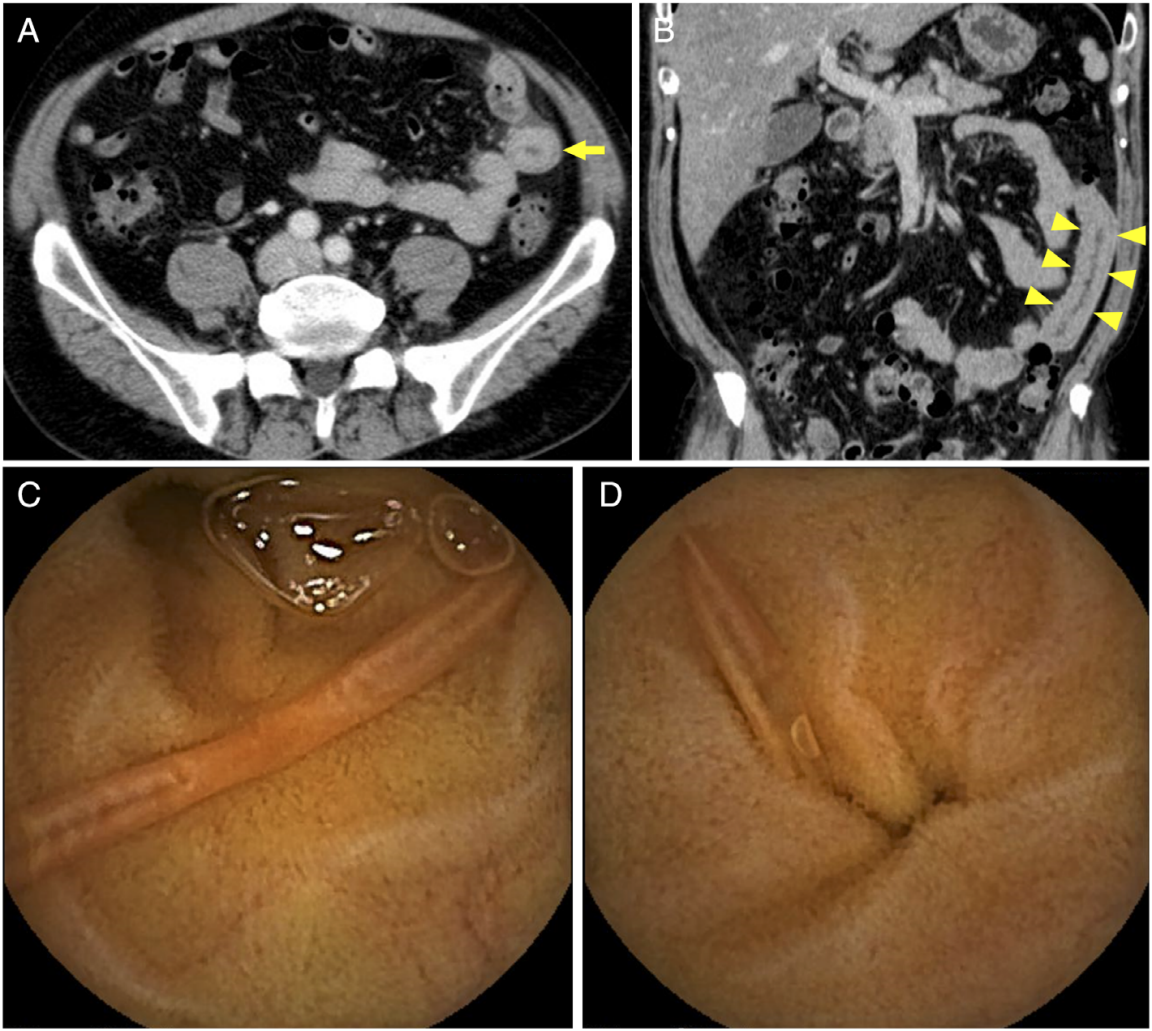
(a) Axial and (b) coronal views of computed tomography showing bowel wall thickening in the jejunum (arrow and arrow heads). (c, d) Capsule endoscopy detected a substantial number of intestinal parasites in the jejunum.
